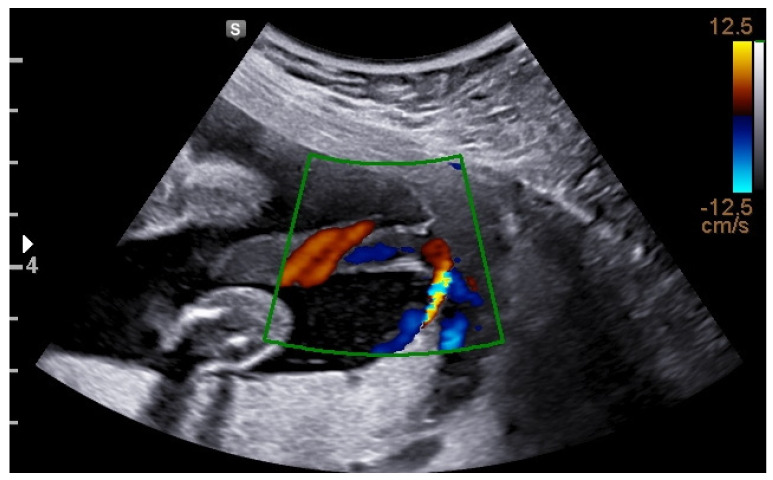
Ultrasound image depicting a velamentous cord insertion.
